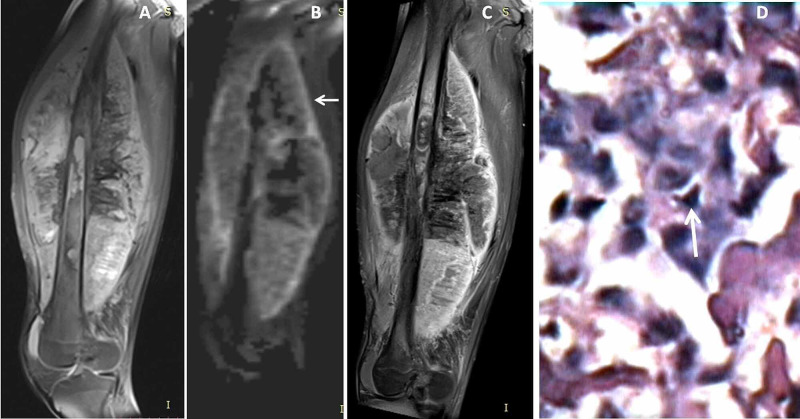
Diaphyseal osteosarcoma Eighteen-year-old male with right thigh swelling. Sagittal proton density fat-suppressed (PDFS) image (A) showed a large lobulated mixed signal intensity mass lesion around the femoral diaphysis with intramedullary altered signal intensities and hypointense sunray appearing new bone formations. Sagittal diffusion-weighted imaging (DWI) (B) showed peripheral dominant diffusion restriction (←arrow). Fat-suppressed post gadolinium sagittal T1 weighted (T1W) image (C) showed heterogeneous enhancement of the mass with areas of necrosis and sunray appearing new bone formation had adjacent soft tissue infiltrations. 40X HPE image (D) showed pleomorphic cells (↑ arrow) with osteoid formations suggesting osteosarcoma.
